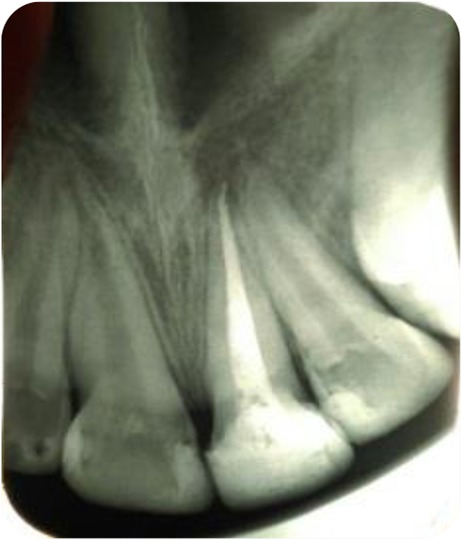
Periapical radiograph at 12-month follow-up
